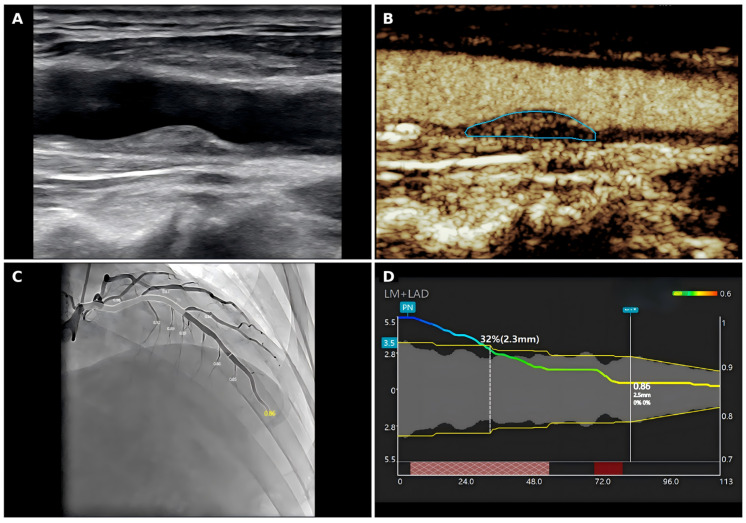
Representative carotid ultrasound, carotid CEUS, and QFR images in a patient with functionally significant coronary artery stenosis. (A) Conventional carotid ultrasound showing carotid plaque morphology. (B) Carotid contrast-enhanced ultrasound showing plaque enhancement and the selected plaque region. (C) Coronary angiography image of the LAD. (D) QFR analysis curve showing the corresponding functional assessment. CEUS, contrast-enhanced ultrasound; LAD, left anterior descending artery; QFR, quantitative flow ratio.
